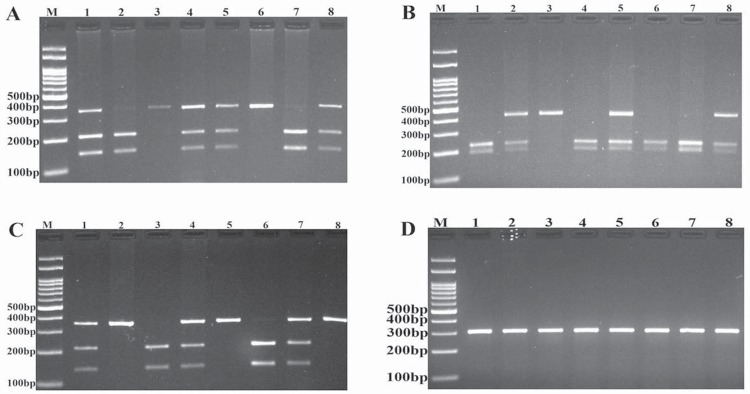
Photograph of electrophoresis pattern of the PCR-RFLP method for detection of AXIN2 rs7224837 (A), BMP4 rs17563 (B), IRF6 rs861019 (C) and IRF6 2235371 (D). M: DNA marker. For AXIN2 rs7224837 variant, lanes 1, 4, 5, and 8: AG; lanes 2, and 7: AA; lanes 3, and 6: AA. For BMP4 rs17563 polymorphism, lanes 1, 4, 6, and 7: TT; lanes 2, 5, and 8: CT; lane 3: CC. For IRF6 rs861019 variant, lanes 1, 4, and 7: GA; lanes 2, 5, and 8: GG; lanes 3, and 6: AA. Regarding IRF6 2235371 variants, all lanes are CC genotype
